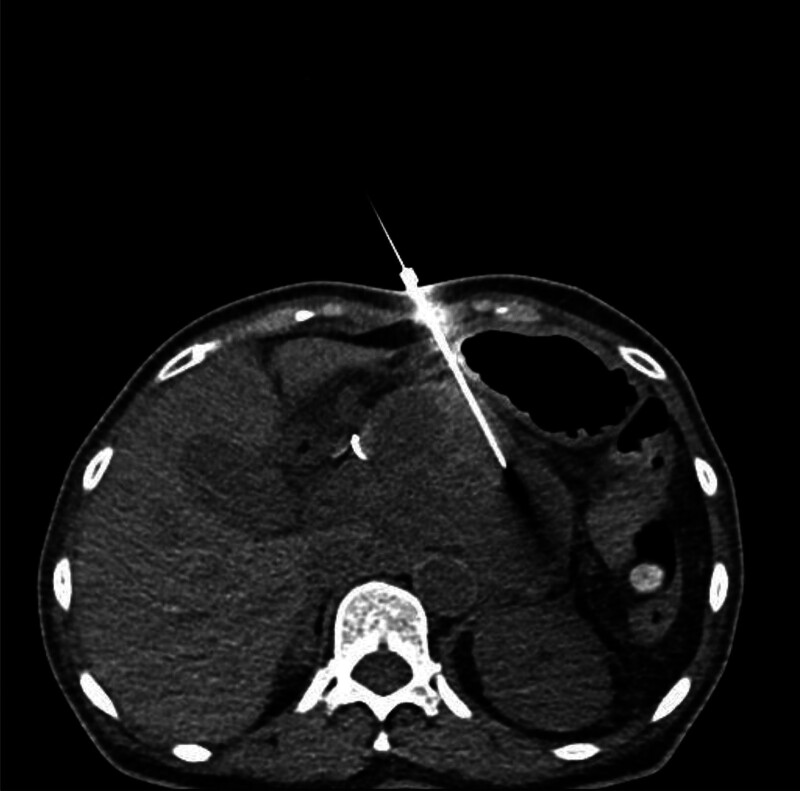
A 19-gauge needle guided by real-time CT imaging, with a puncture path from the outside of the lesion into the pseudocyst.
